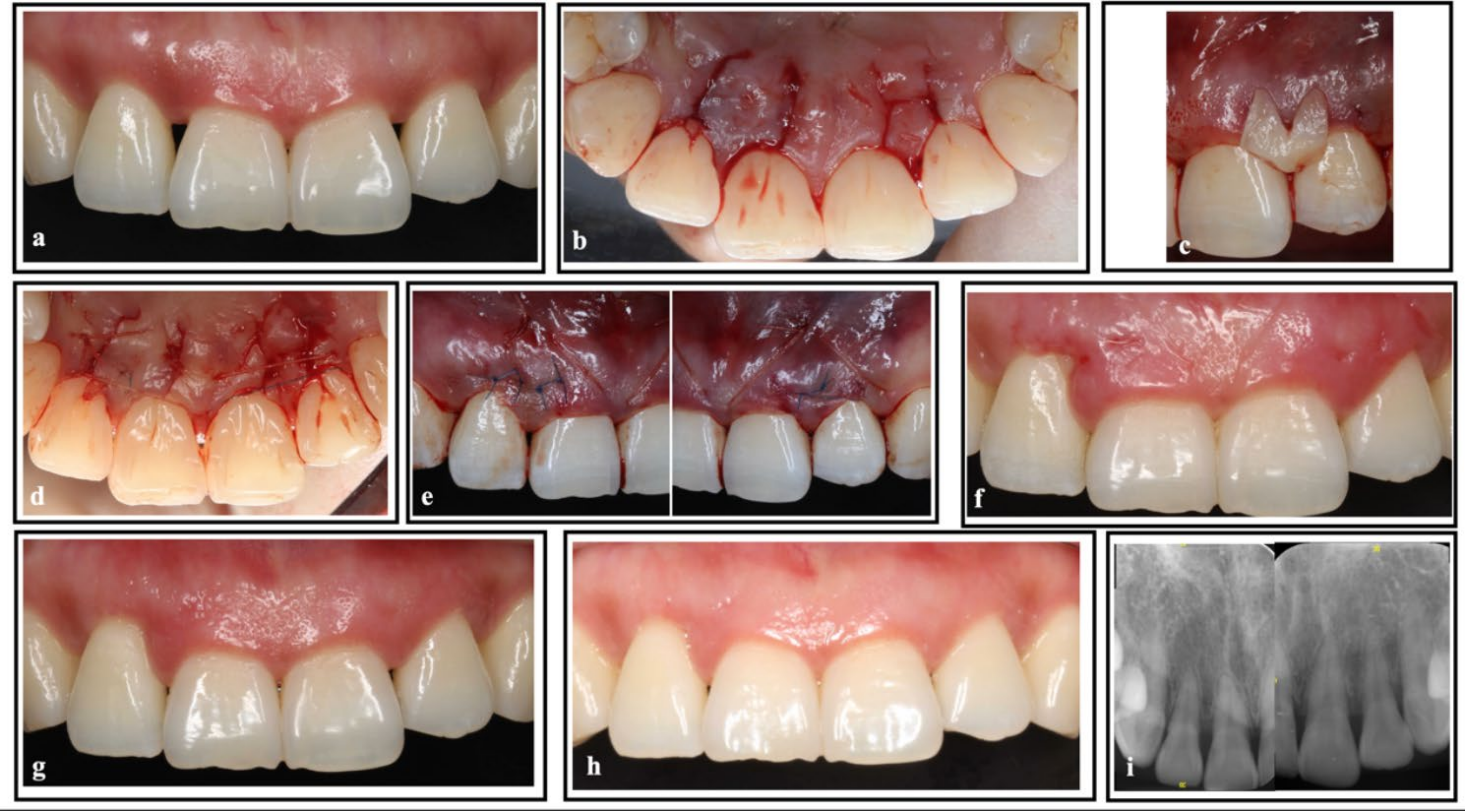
Surgical procedure and follow-up of Case 1. (a) Preoperative view showing the defects in the papilla between the right central and lateral incisors and between the left central and lateral incisors. (b) Vertical incision and cutback on the palatal side. (c) A CTG with a special shape. (d) Postoperative view after suturing at the palatal side. (e) Postoperative view after suturing at the labial side. (f) Suture removal 2 weeks after surgery. (g) At 3 months post-MIPT surgery, the height of the gingival papilla was restored. (h) At 12 months post-MIPT surgery, the gingival papilla in the surgery area was almost filled. (i) Radiographic image at baseline CTG, connective tissue graft; MIPT, modified interproximal tunneling
